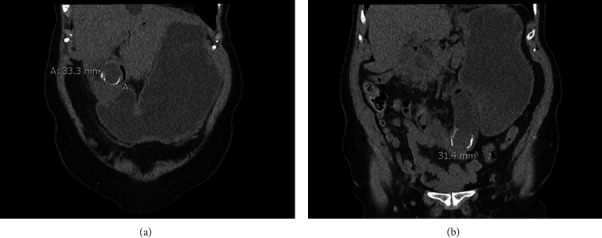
(a) Abdominal CT scan showing a 33-mm stone causing gastric outlet occlusion. (b) A 31-mm stone causing obstruction of the proximal jejunum.
